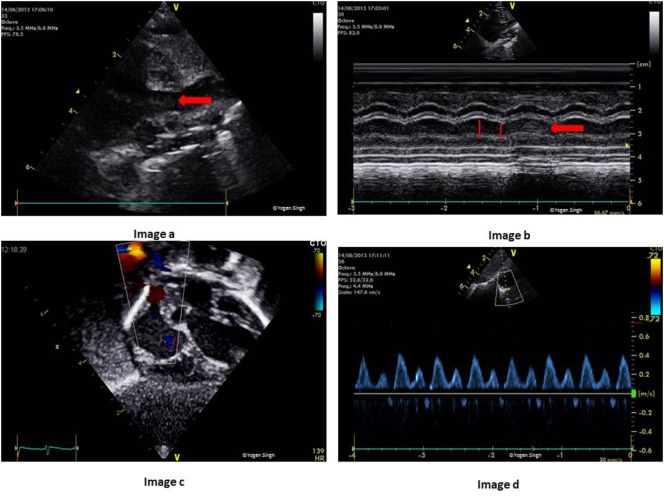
Measurement of superior vena cava (SVC) flow on echocardiography. Image a shows SVC in modified parasternal short axis view on 2D and image b with measurement of SVC diameter in M-mode. Image c shows acquisition of pulse-wave Doppler in the sub-costal view and SVC flow in milliliters per minute has been calculated in image d after measuring velocity time integral.
